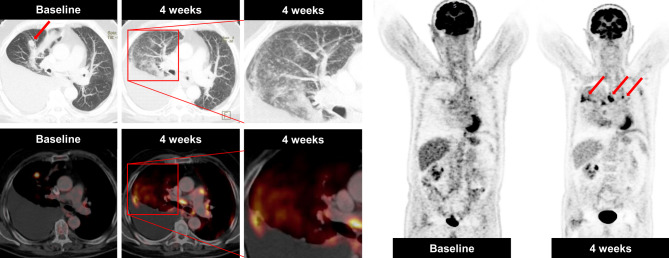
A case of a sarcoid-like reaction in a 76-year-old male NSCLC patient, who was receiving immune checkpoint inhibitor therapy. Axial CT in lung window and fused ^18^F‑FDG-PET/CT imaging obtained before treatment initiation showed a metabolically active metastasis in the middle lobe and a right pleural effusion. Four weeks after treatment initiation, multiple intrapulmonary micronodules in a perilymphatic distribution were detectable in both lungs, predominately right ≫ left. In addition, enlarged metabolically active hilar/mediastinal lymph nodes were visible (*arrows*), consistent with a sarcoid-like reaction. The disappearance of the metastasis in the middle lobe was suggestive of treatment response
